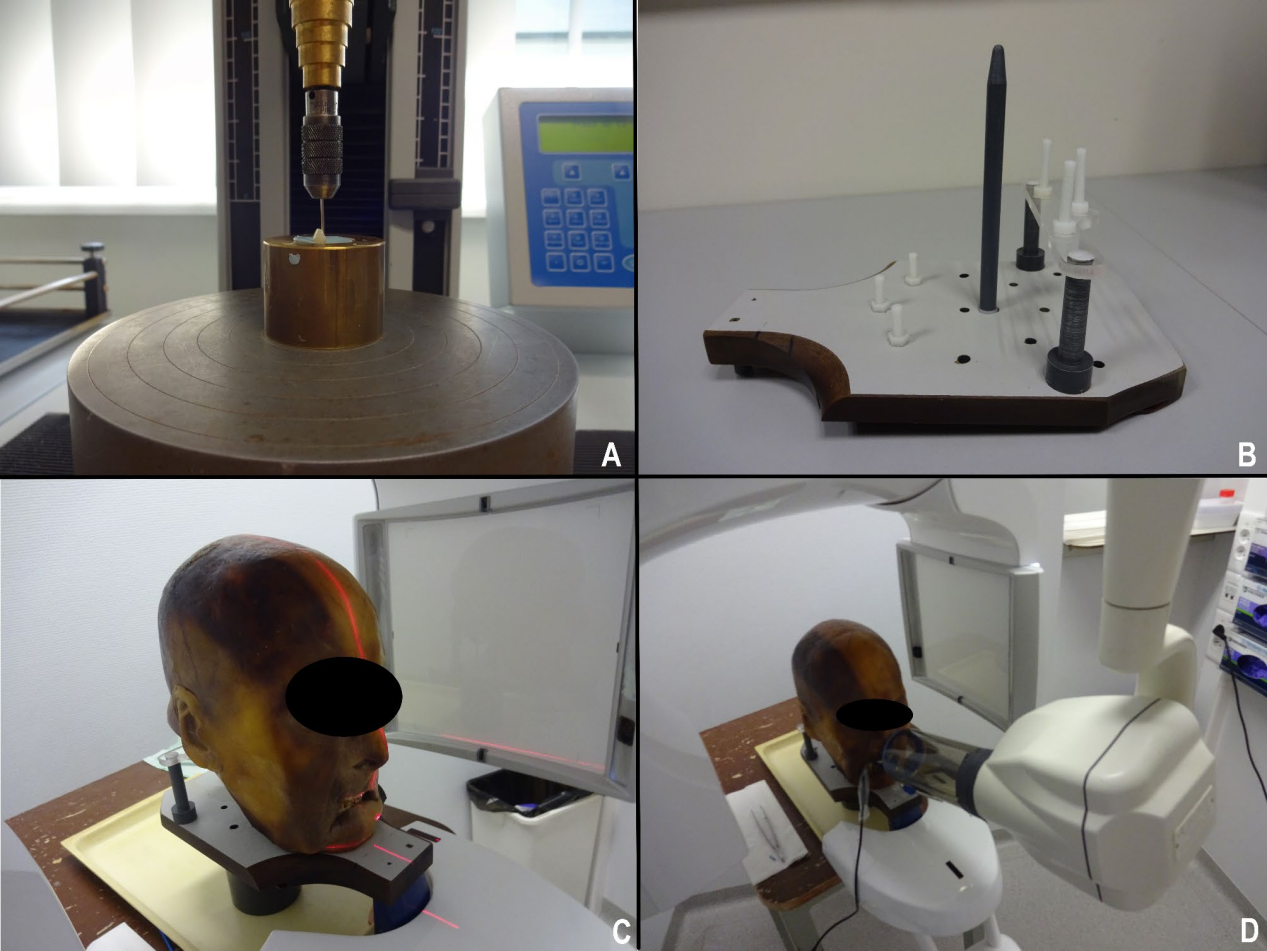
Material and methods: (a) Fracture initiation. (b) Custom platform for skull fixation. (c) CBCT imaging of the samples. (d) Periapical imaging of the samples
